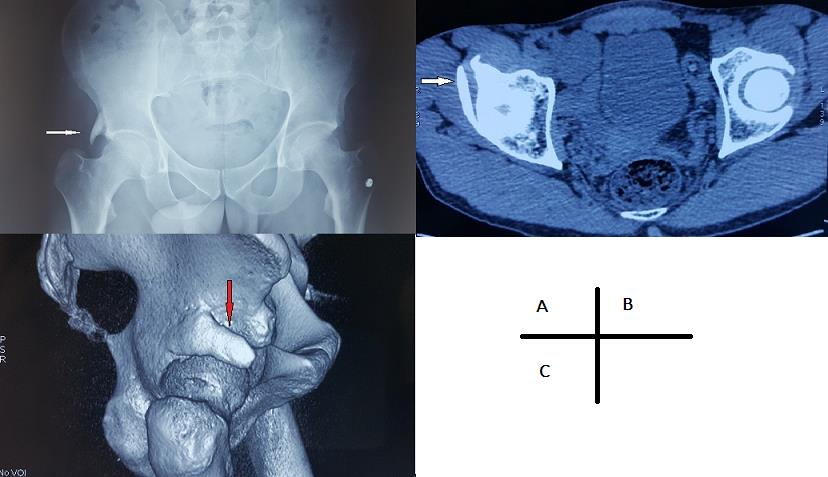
A) radiographie standard du bassin face montrant une protubérance osseuse en forme de doigt; B) tomodensitométrie en coupe axiale montrant le doigt pelvien s’articulant avec le toit du cotyle droit; C) tomodensitométrie avec reconstruction 3D montrant le doigt pelvien s’étendant en direction antéro-inférieure
